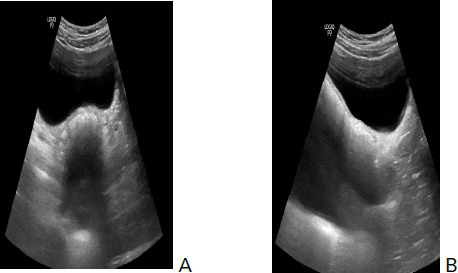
Axial and sagittal ultrasound images showing absence of uterus in pelvic cavity (A) and (B).
